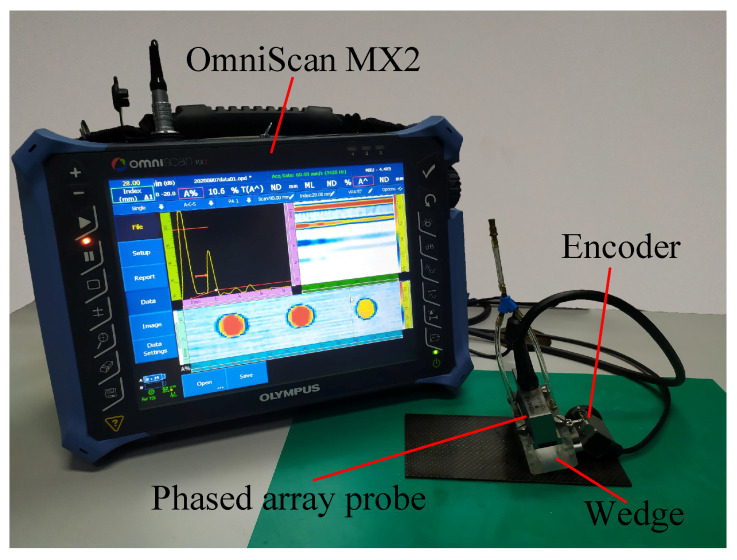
Phased array ultrasonic testing system.
